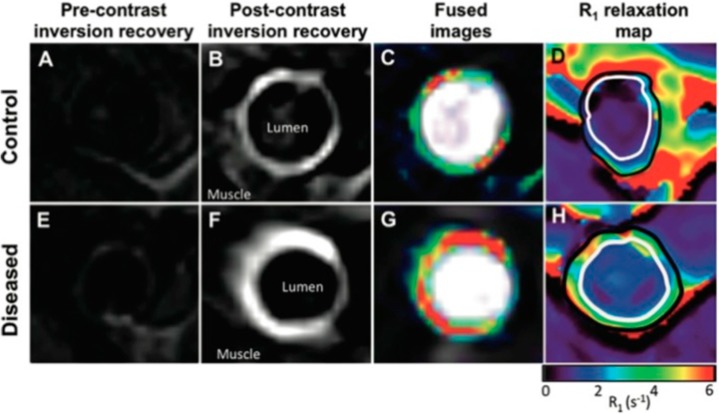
MRI of Aortic Cross-sections Show Higher Uptake of Elastin-Specific Contrast Agent and R1 Relaxation Values in Atherosclerotic Compared with Control Aortas. (A,E) Phase-contrast MRA pre-injection and (B,F) delayed-enhancement (D,E) MRI post-injection of elastin-specific contrast agent; (C,G) DE MRI fused with phase-contrast angiograms; (D,H) Corresponding R1 relaxation maps post-injection of elastin-specific contrast agent showing less relaxation in the atherosclerotic aorta. Reproduced with permission from *Phinikaridou et al.* [139].
